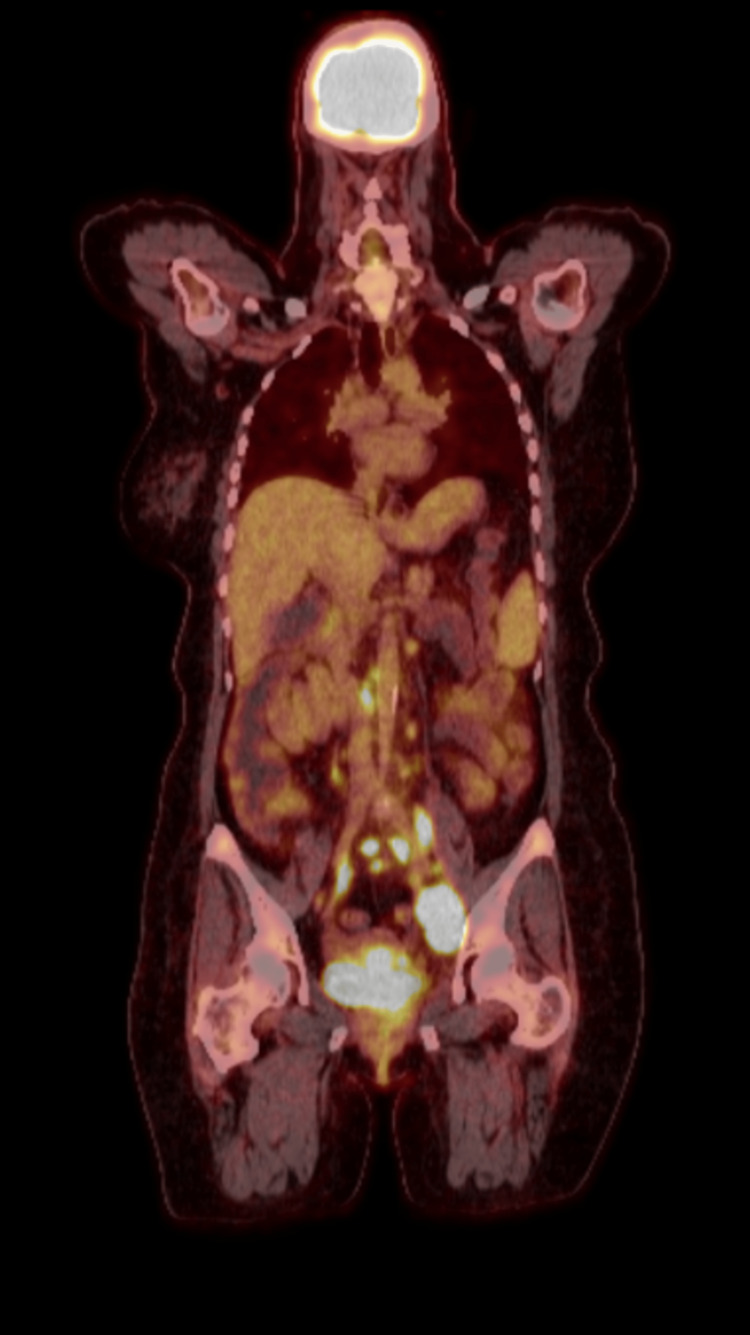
Coronal positron emission tomography (PET) scan and a computed tomography (CT) scan of the skull to thigh. Coronal view of fluorodeoxyglucose (FDG)-positron emission tomography (PET) scan of the patient showed FDG uptake in the cervix and multiple pelvic and para-aortic lymph nodes with extension to renal vasculature.
